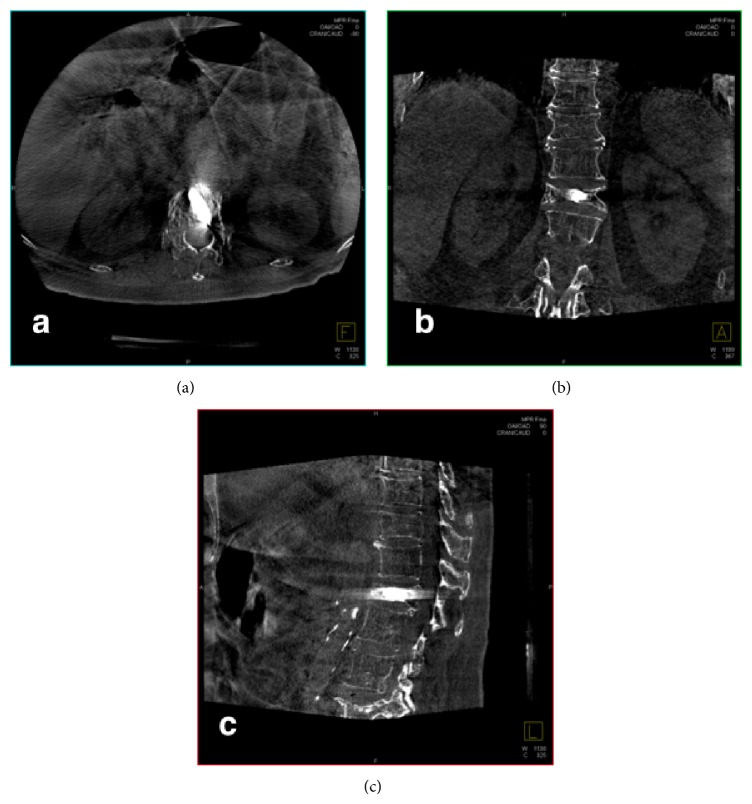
Flat panel detector-CT native images allow CT-like visualization of the disposal of intravertebral cement and its relation to neural foramina and the spinal canal ((a) and (c)).
